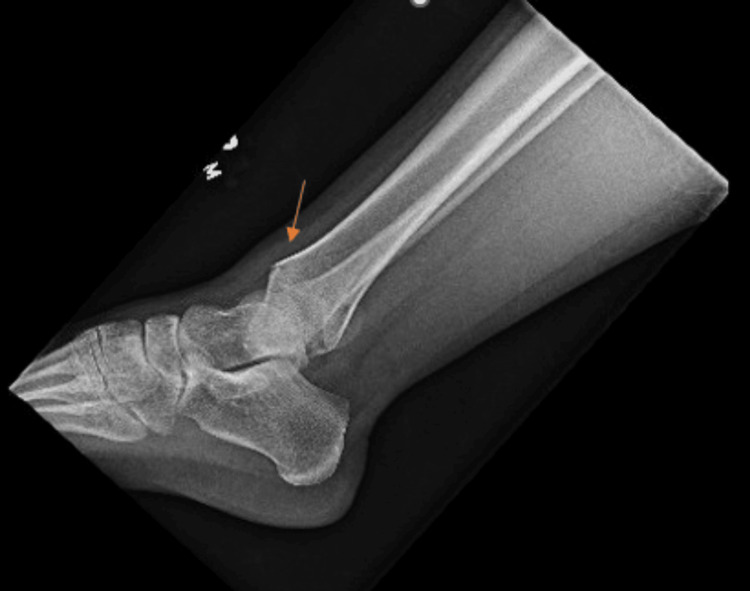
Lateral radiograph of the ankle X-ray series The orange arrow shows a nondisplaced fracture of the distal tibial metadiaphysis with extension to the articular surface laterally
